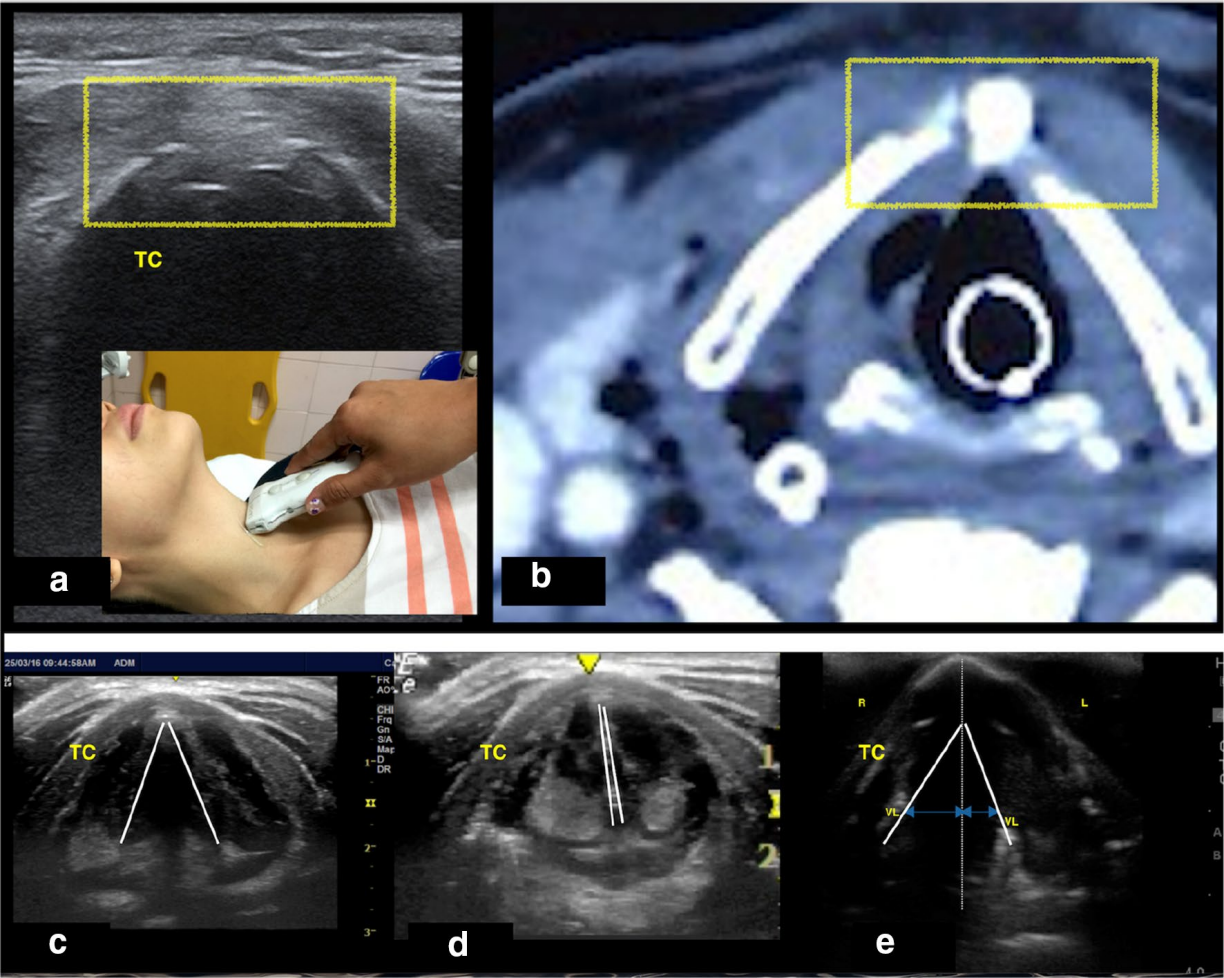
a Image of focused airway of displaced thyroid cartilage fracture and disruption of anterior cortex of the thyroid cartilage (box). b A computerized tomography (CT) scan image showing defect in posterolateral wall of trachea with fracture of right anterior lamina of thyroid cartilage and superior cornu of left thyroid cartilage. c, d Assessment of vocal cord mobility can be done by looking at the movement of vocal ligament (white line) during abduction and adduction. e Blue arrow indicates reduced movement of the right vocal ligament (white line) to the midline during adduction compare to the left vocal ligament. R: right; L: left; VL: vocal ligament, TC: thyroid cartilage
